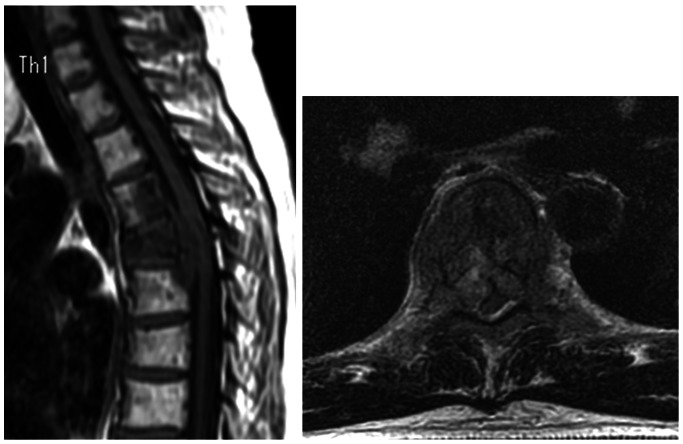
Preoperative MRI. This patient had metastasis invading into the spinal canal at the T5 level.
